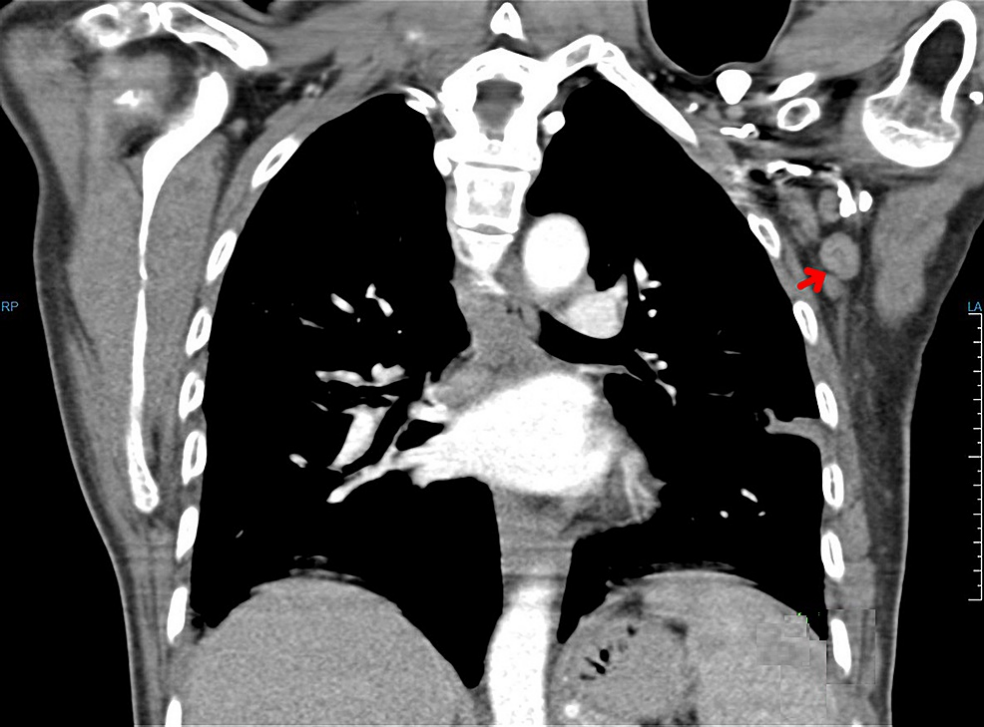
Chest CT (May 22, 2016) showing enlarged left axillary lymph node of 1.3 cm
